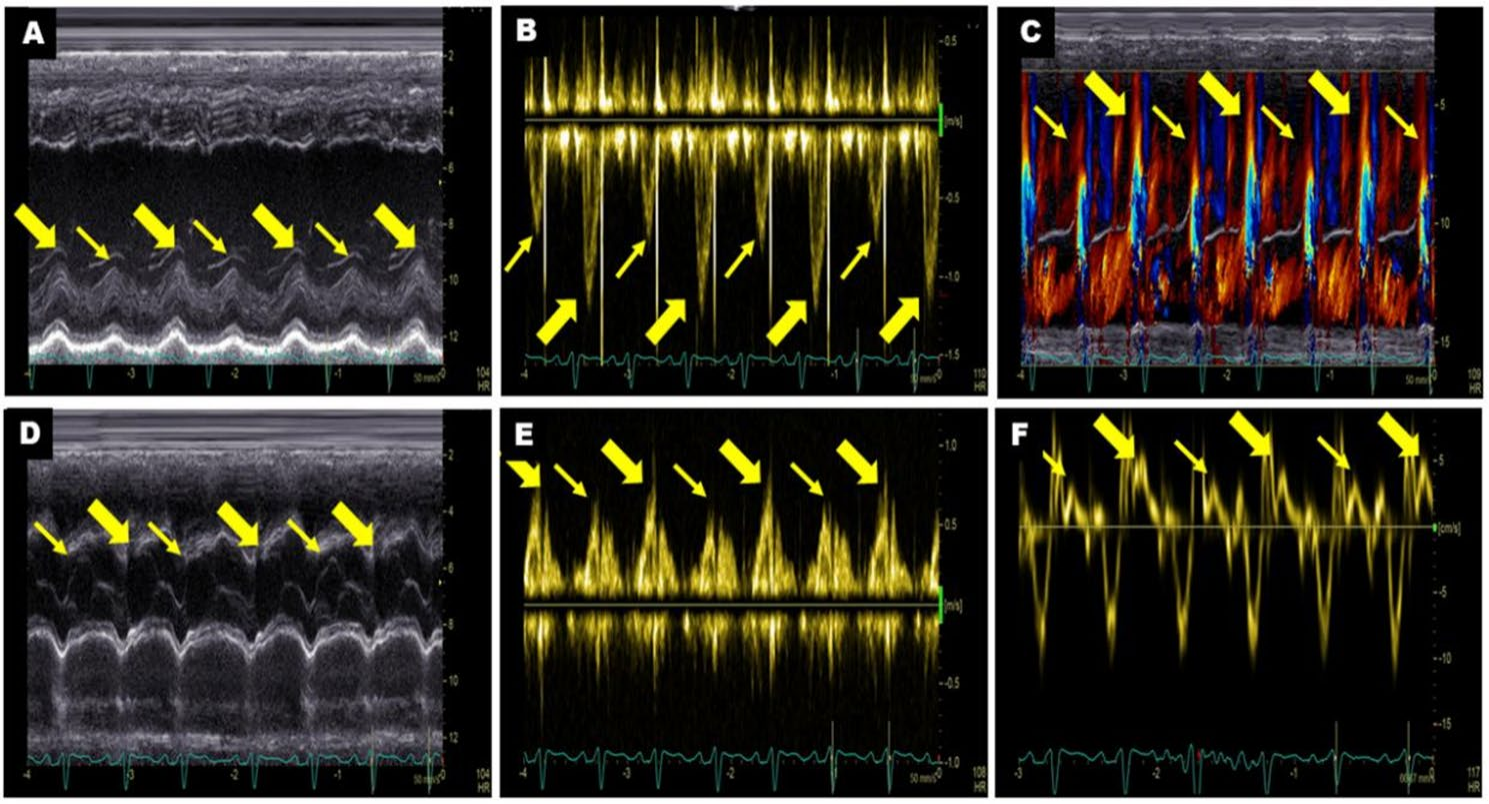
Multiple echocardiographic images of a patient with left ventricular pulsus alternans. A M-mode echocardiography showing beat-to-beat alternations in the left ventricular inner diameter. B Pulse-wave Doppler demonstrating beat-to-beat alternations of left ventricular outflow tract velocities. C Colour M-mode Doppler showing beat-to-beat alternations of diastolic transmitral flow. D M-mode echocardiography showing beat-to-beat alternations in aortic valve excursion during systole. E Pulse-wave Doppler showing beat-to-beat alternations of diastolic mitral valve flow velocities. F Tissue Doppler showing beat-to-beat alternations of mitral annulus systolic S′ velocities. The bold yellow arrow represents a strong beat, and the thin yellow arrow represents a weak beat on different echocardiographic images
